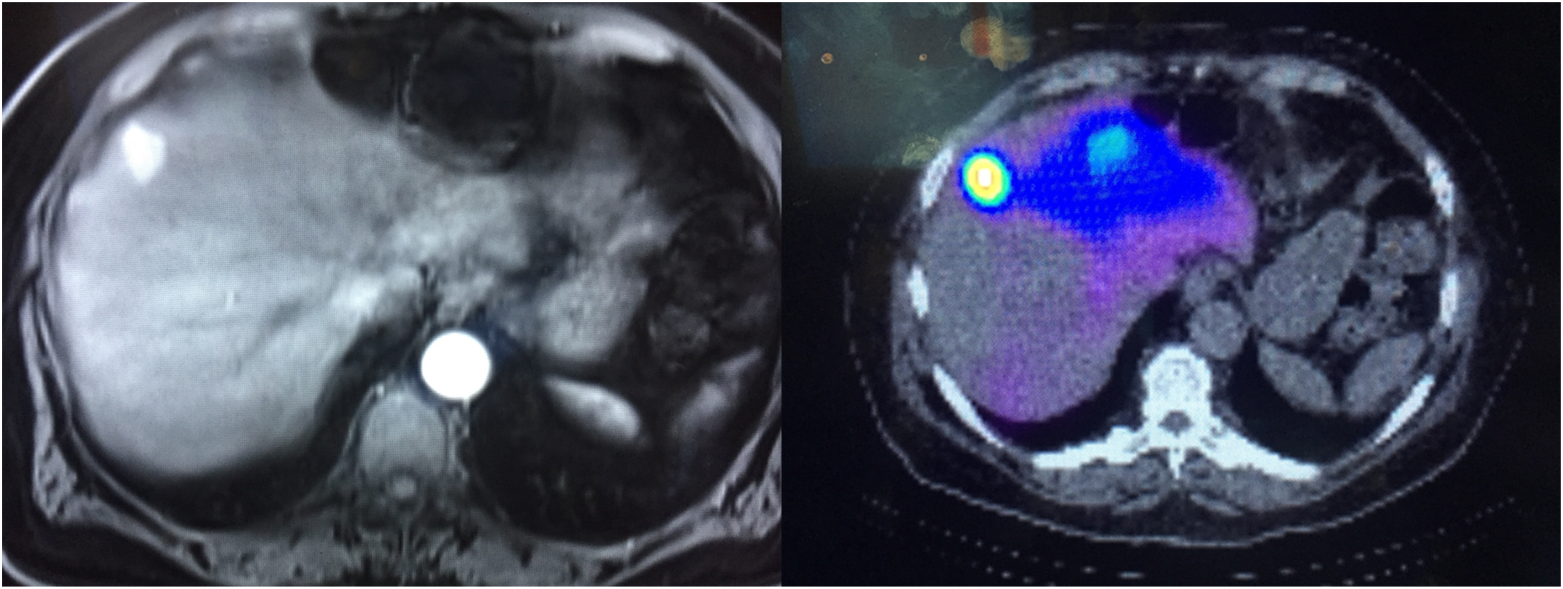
Left shows a contrast-enhanced CT of the renal cell carcinoma lesion being treated. Right shows a post-therapy fused non-contrast CT and bremmstrahlung SPECT.
